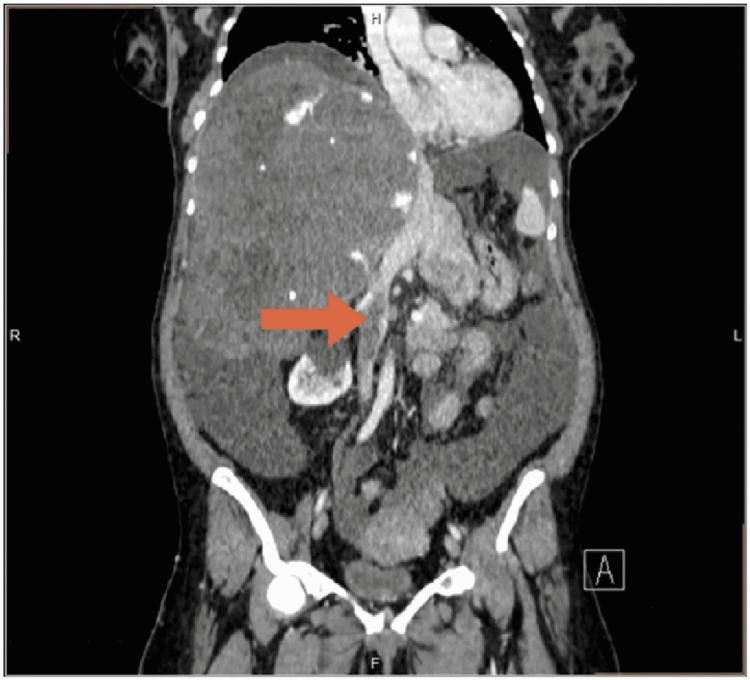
Coronal non-contrasted CT image showing the enlarged liver measuring 19 x 15 x 22 cm in cranial-caudal diameter and the IVC thrombus measuring 5.7 cm (arrow).
